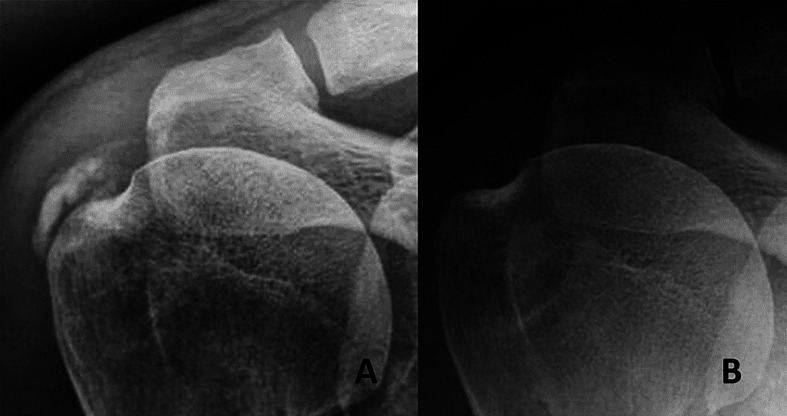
(A) AP view of a right subacromial space showing a Gärtner type II calcification located in the supraspinatus tendon. (B) The same X-ray projection after three sessions of focused shock waves.
